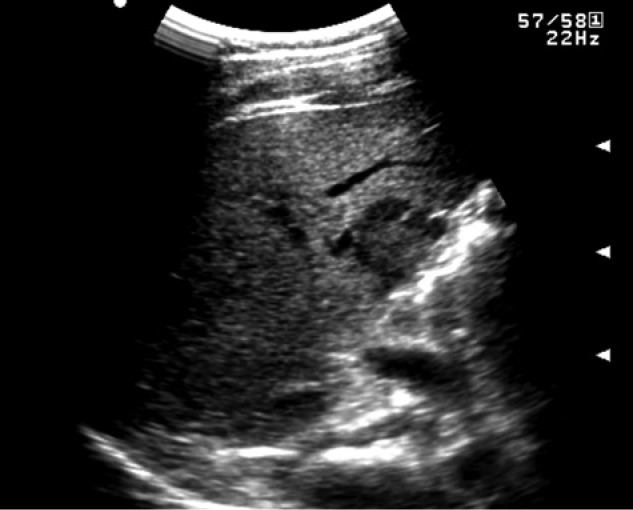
Abdominal ultrasonography image of a 22-year-old man without underlying liver disease shows a well-defined, inhomogeneous echoic hepatic nodule with a predominantly hypoechoic rim in the periphery of the right hepatic lobe.
